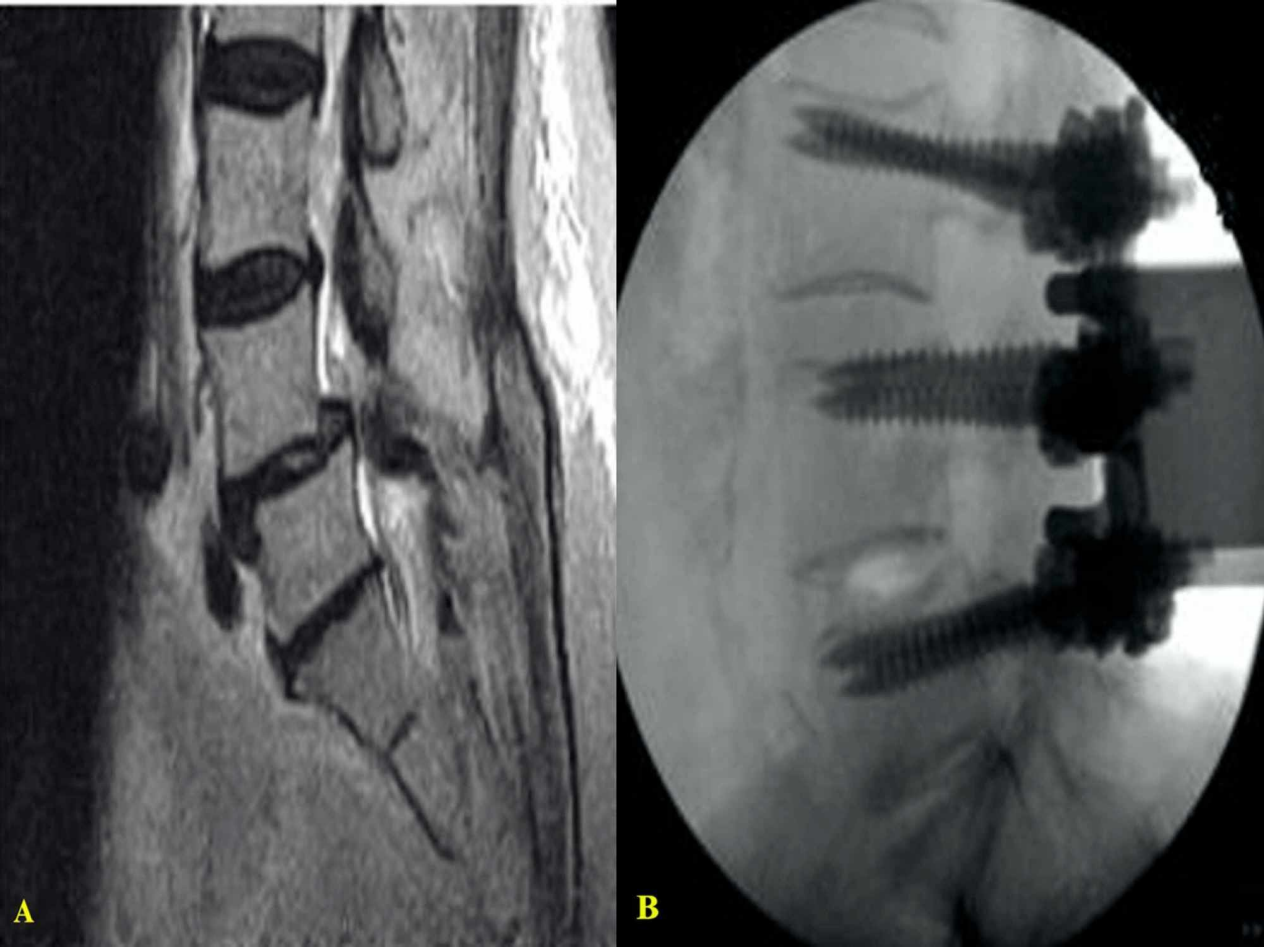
(A) CT lumbar spine (sagittal view) shows severe spinal stenosis due to L4-5 anterolisthesis. (B) Intraoperative X-ray (lateral view) shows the correction achieved with the UNIMAX pedicle screw-plate system
